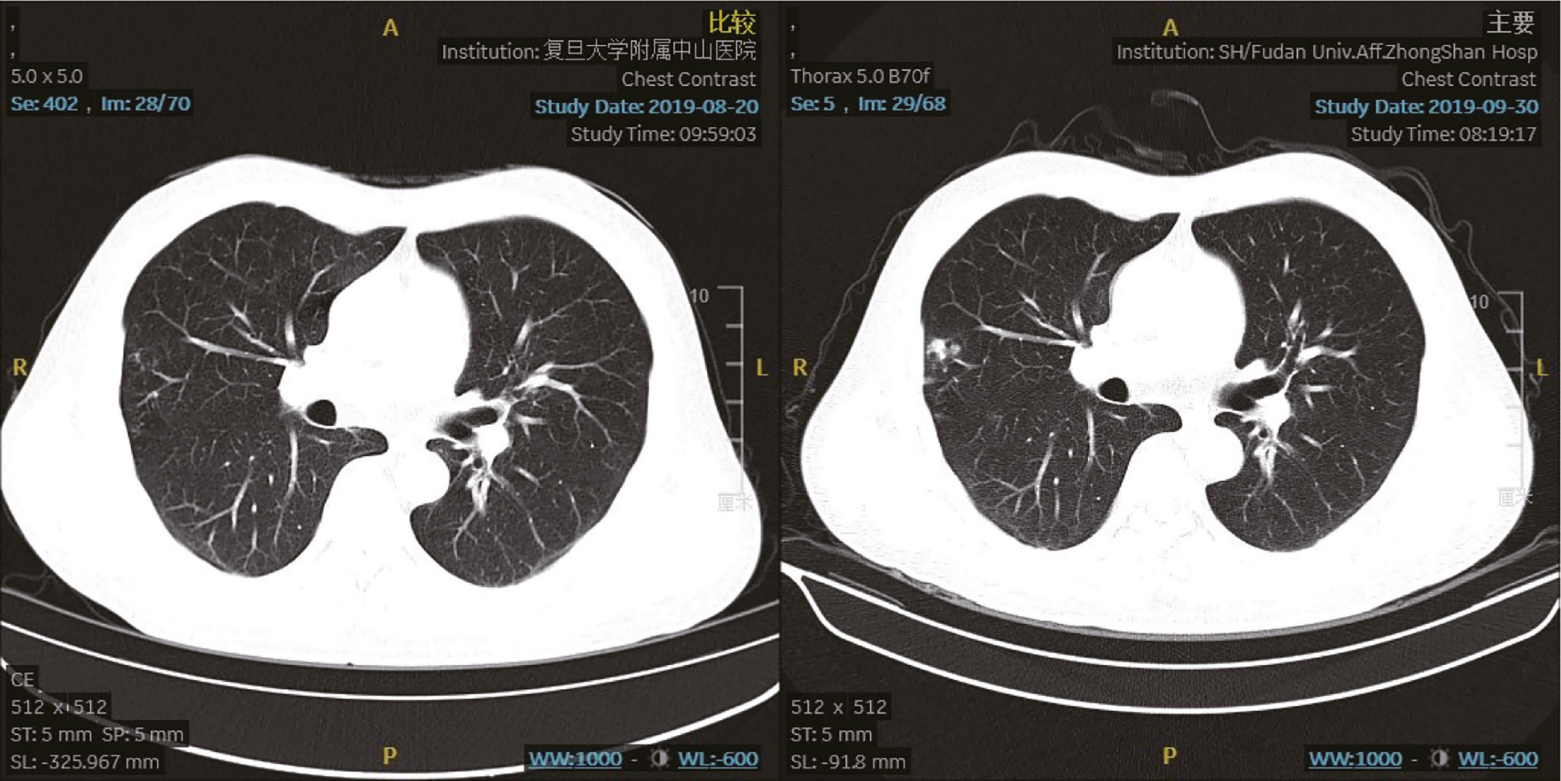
Chest computed tomography scan. The chest computed tomography lung window (left picture 20 August 2019; right picture 30 September 2019) shows new nodules on the lateral edge of the right lung, which may suggest inflammatory.
